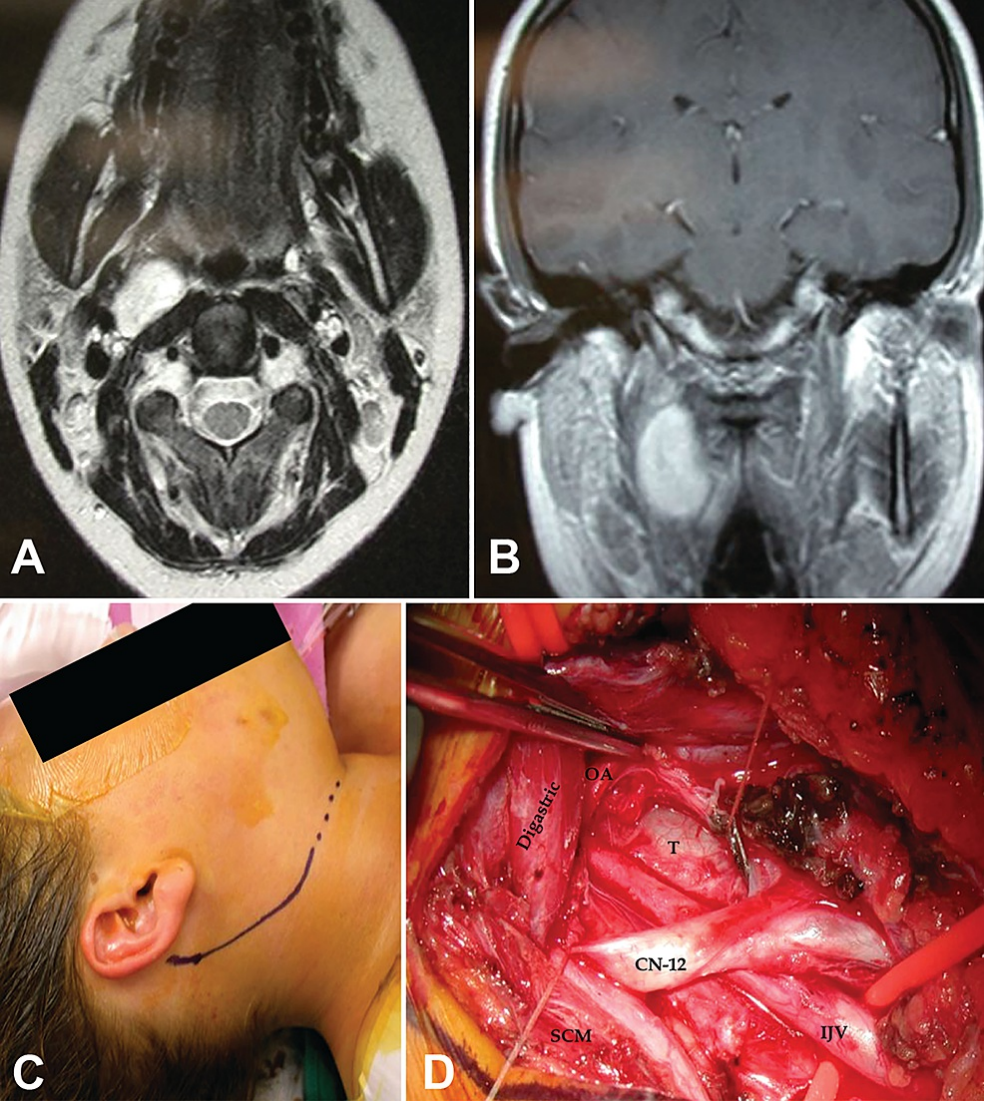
Glossopharyngeal schwannoma accessed via stylodigastric triangle. Axial T2 (A) and coronal T1 (B) gadolinium-enhanced MRI of a glossopharyngeal schwannoma in a pediatric patient with neurofibromatosis type-1 presenting with a neck mass (case 4). Note the tumor’s location is distal in the neck and medial to the internal carotid artery. A distal lateral cervical exposure was performed (C, D) and the stylodigastric triangle opened by mobilizing the digastric muscle. Labels: CN-12: hypoglossal nerve; IJV: internal jugular vein; OA: occipital artery; SCM: sternocleidomastoid muscle; T: tumor.
